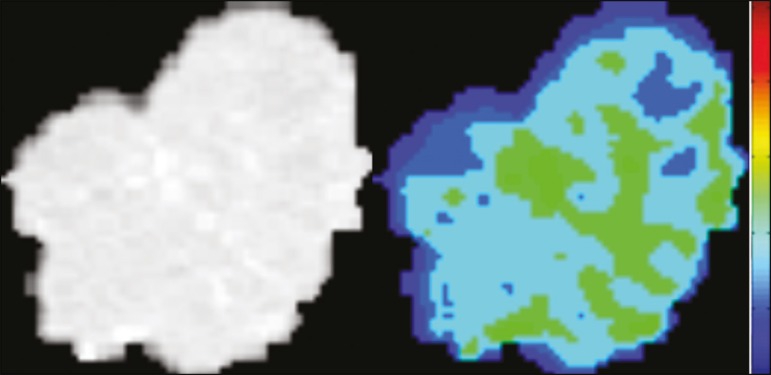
Quantification of the heterogeneity of a pulmonary adenocarcinoma on a CT scan of the chest by radiomic/radiogenomic evaluation. Color scale refers to a feature extracted from the image, reflecting tissue and genetic subregions of the tumor.
